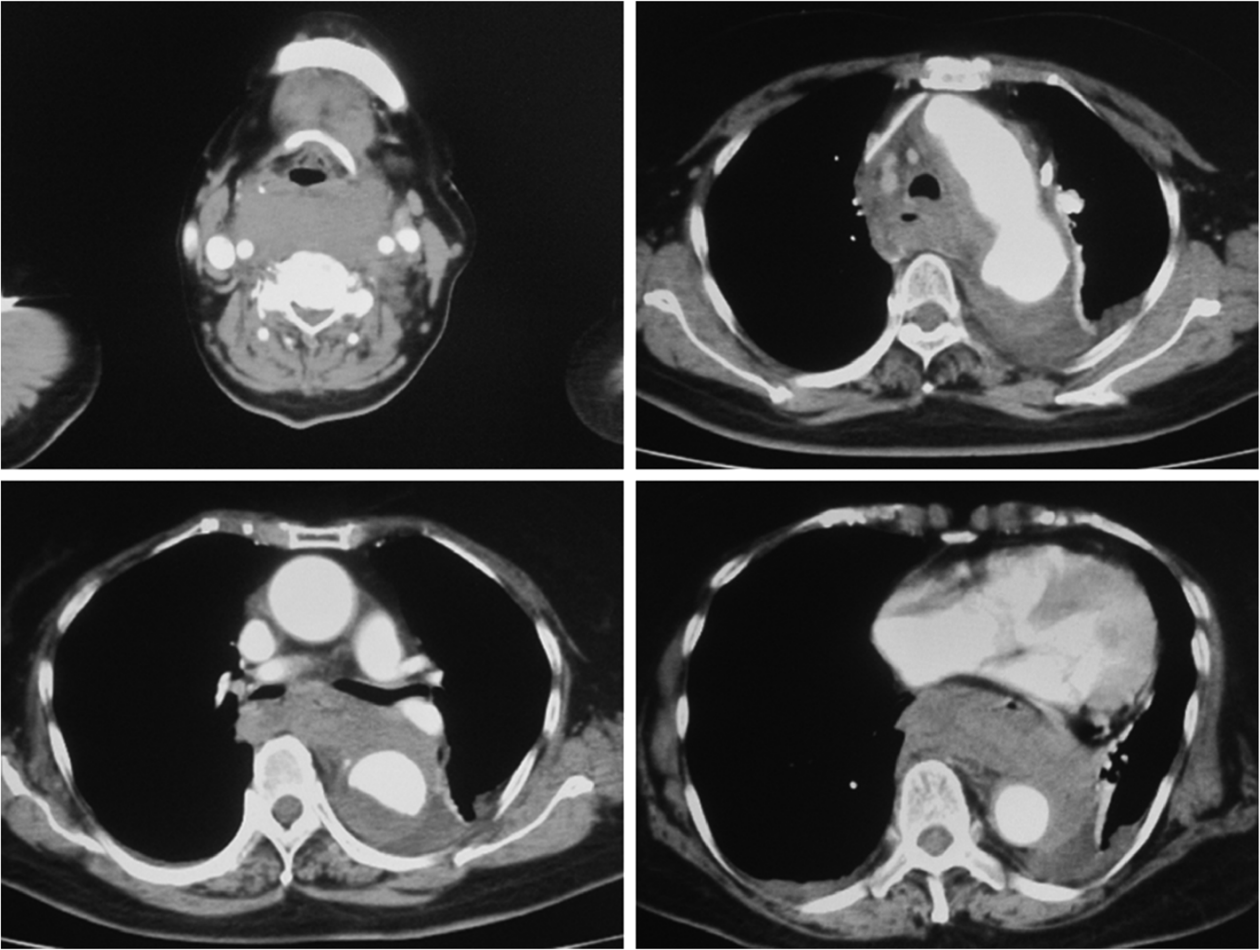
Contrast-enhanced computed tomography scans. The larynx was compressed and narrowed by the hematoma. A large cervico-mediastinal hematoma was detected.
